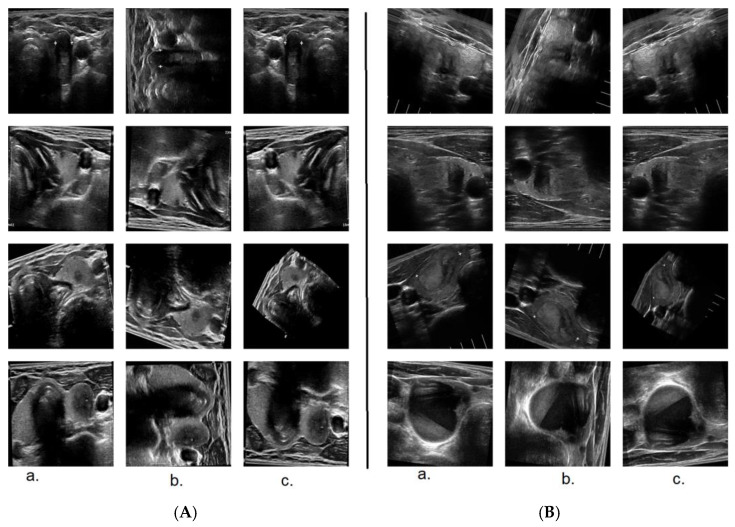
Representative ultrasound images of thyroid cancer and normal thyroid with corresponding data augmentation transformations. The first three columns display original ultrasound images of thyroid cancer. (A) Data augmentation technique: Number of images of a thyroid cancer class. (a), alongside augmented variants (b,c). The following three columns present original ultrasound images of normal thyroid tissue. (B) Data augmentation technique: Number of images of a normal thyroid class. (a), with their corresponding augmented images (b,c) generated through data augmentation techniques.
